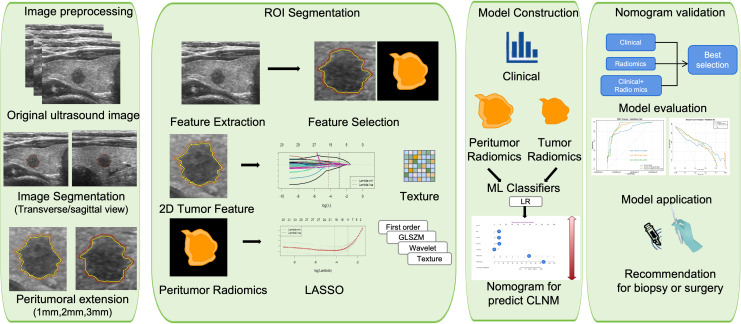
Workflow of the lymph node metastasis (LNM) prediction system. (I) Manual segmentation of thyroid ultrasound images in transverse and sagittal views, including peritumoral extension. (II) Extraction of radiomics features from the region of interest (ROI), including texture, first-order, GLSZM, and wavelet features. (III) Feature selection using methods such as LASSO. (IV) Model construction using machine learning classifiers (LR) and integration into a nomogram combined with clinical factors for predicting central lymph node metastasis (CLNM). (V) Model validation and evaluation, supporting clinical recommendations for biopsy or surgery.
